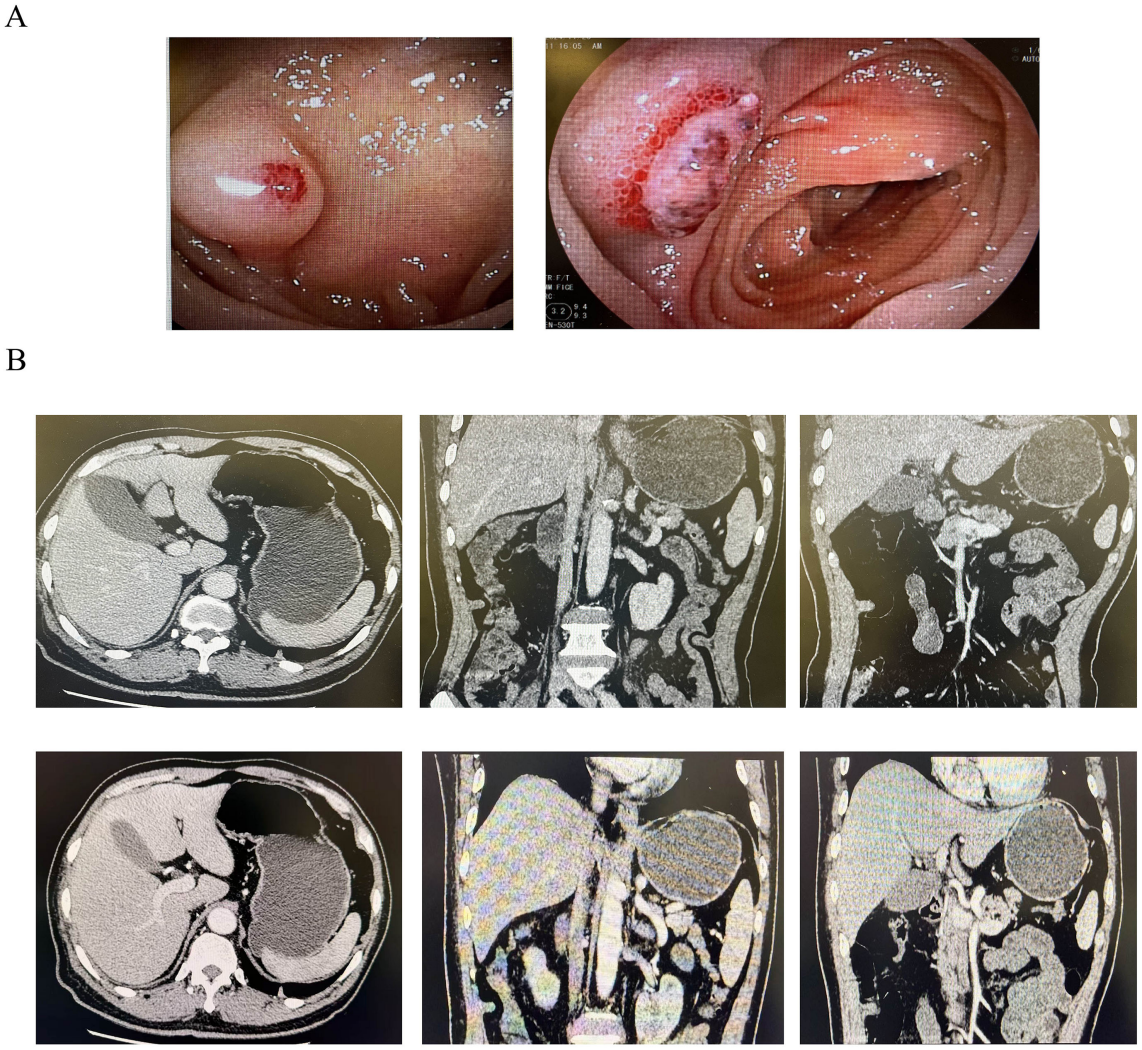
Contrast-enhanced CT and small enteroscopy before surgery. (A) Small enteroscopy showing a bulge with surface mucosal erosion and ulcerative changes, and visible blood vessels are exposed. (B) Plain (*up*) and contrast-enhanced (*down*) CT showing no hemorrhage or occupancy in the digestive system.
